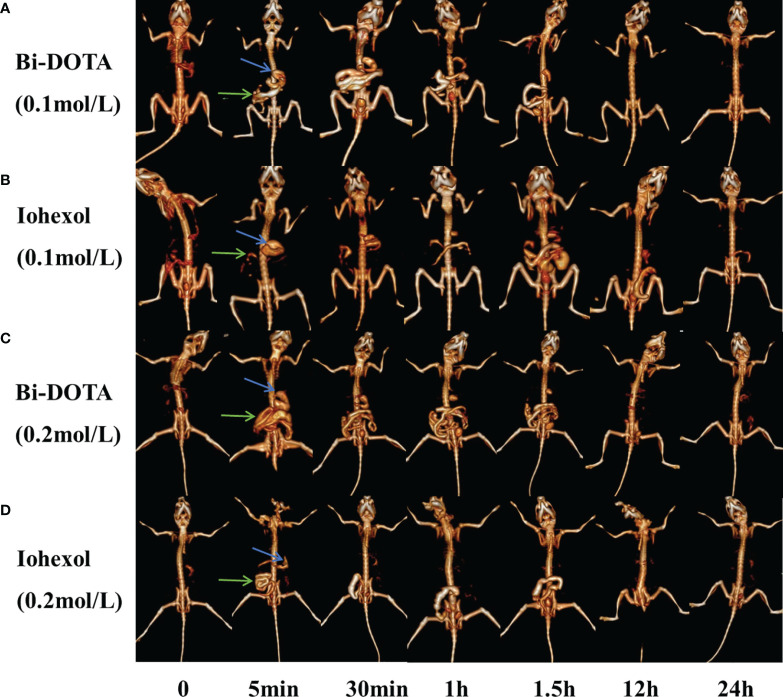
In vivo* GI tract CT imaging after oral administration of (A) 0.1 mol/L Bi-DOTA, (B) 0.1 mol/L iohexol, (C) 0.2 mol/L Bi-DOTA, and (D) 0.2 mol/L iohexol (blue arrows represent stomach and green arrows represent small intestine).
